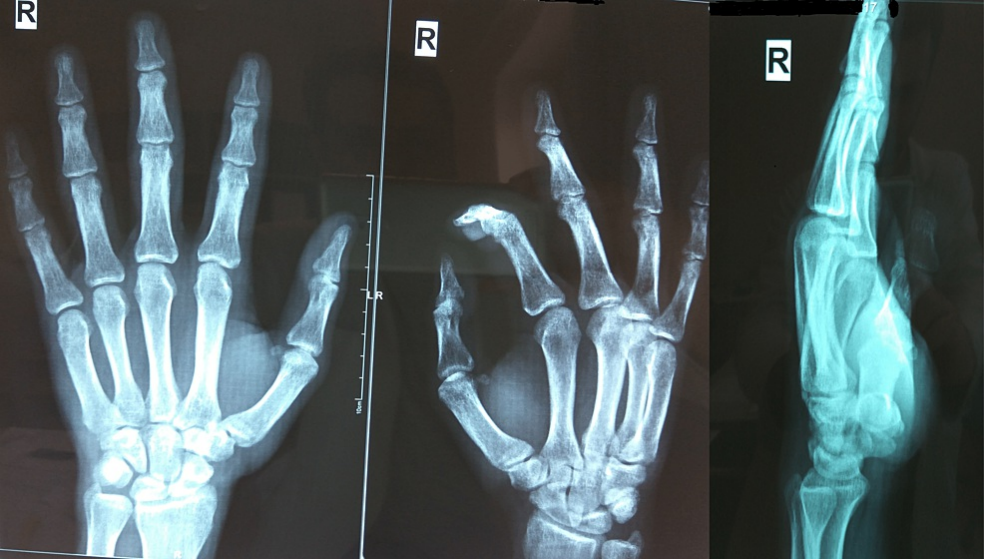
Anteroposterior, oblique, and lateral radiographic views two months after trauma
